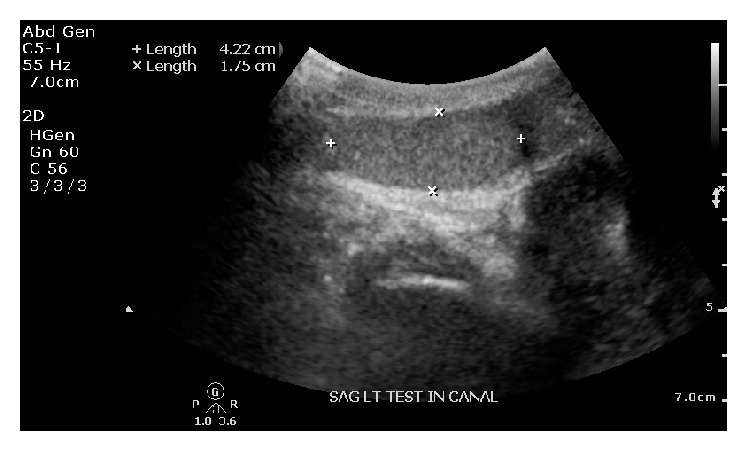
Ultrasound of left testicle in the inguinal canal.
